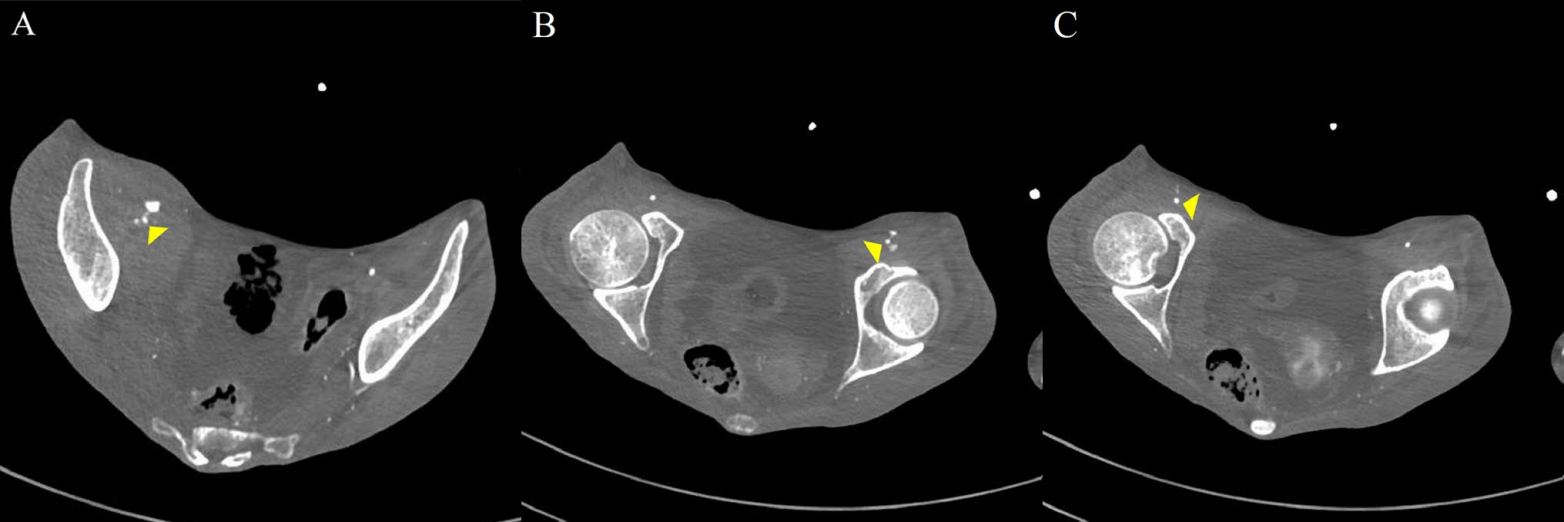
Pelvic contrast-enhanced computed tomography. A Contrast extravasation from the right external iliac artery during the arterial phase (yellow arrow). B Contrast extravasation from the left femoral artery during the arterial phase (yellow arrow). C Contrast extravasation from the right femoral artery during the arterial phase (yellow arrow)
